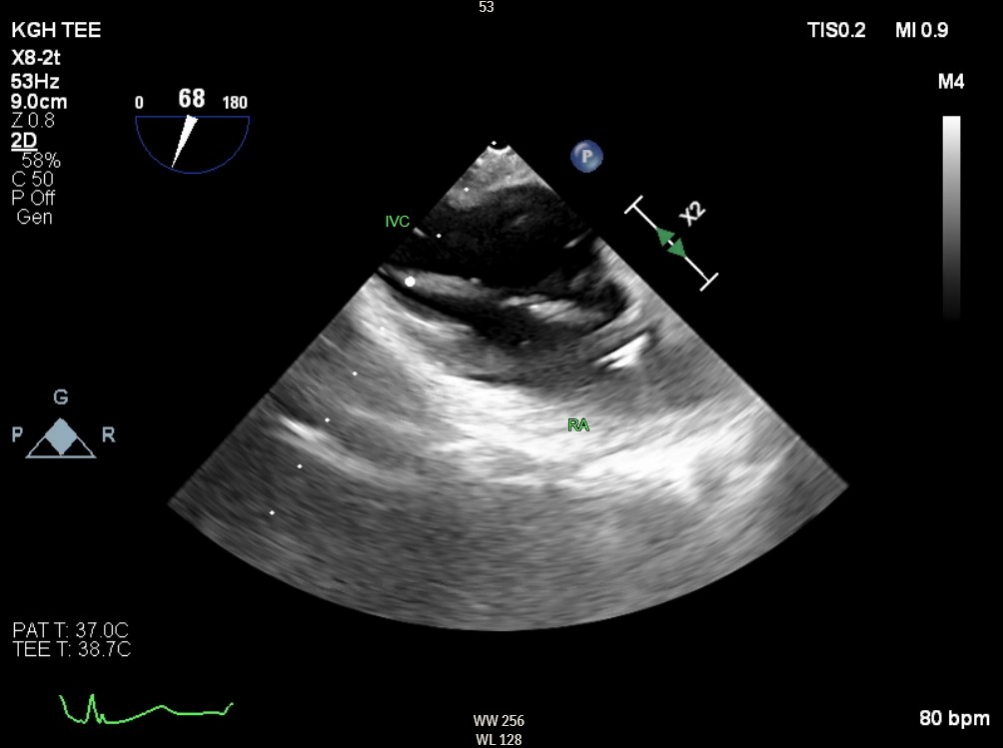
A transesophageal modified bicaval view demonstrating traction on the balloon catheter causing occlusion of the right inferior vena cava orifice.
